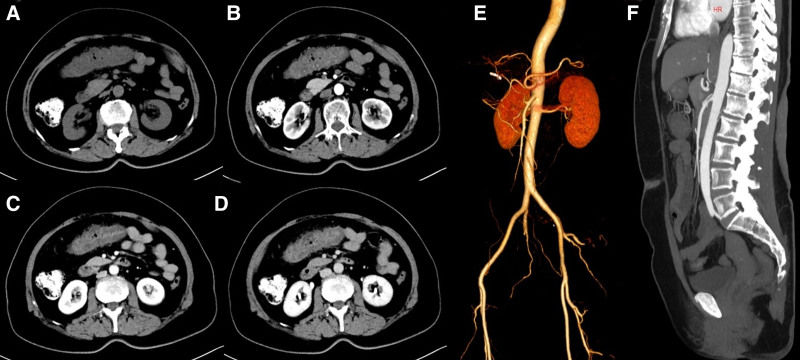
Full abdominal CT plain scan, also known as dynamic contrast-enhanced scan (coronary reconstruction image). (A) Plain scan showing edema and thickening of the transverse colon wall, with blurred fat spaces around it; (B) enhancement, arterial phase; (C) enhancement, portal phase; (D) enhancement, delay period; (B, C and D) dynamic enhanced scanning, no abnormal enhancement observed in the third phase tube wall; (E, F) three-dimensional reconstruction, no intestinal vascular stenosis or thrombosis observed.
